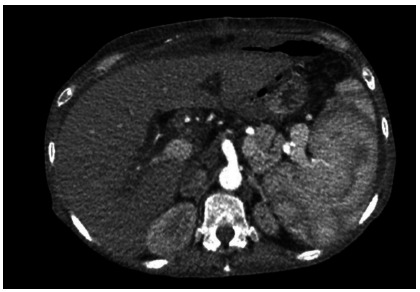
Abdominal computed tomography scan with intravenous contrast at arterial phase showing free air anterior to the stomach, periportal edema of the transplanted liver and splenomegaly.
